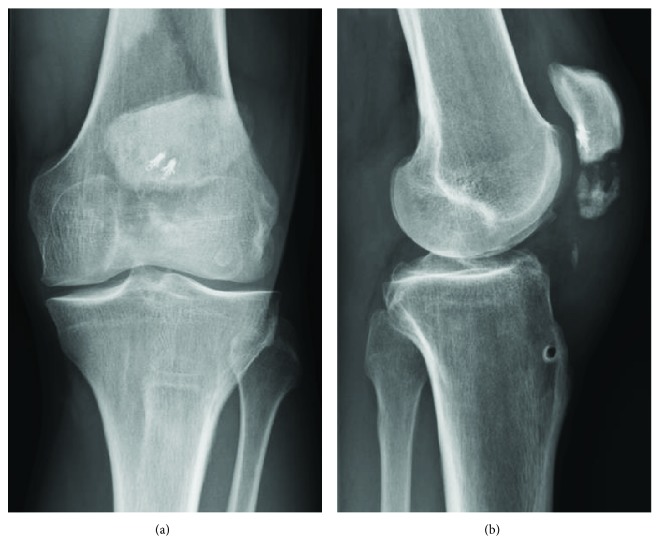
(a) Anteroposterior and (b) sagittal preoperative X-rays before the revision surgery. The iterative rupture of the extensor mechanism is explained by the fracture of the transverse patellar tunnel drilled during the initial surgery to pass the semitendinosus graft.
